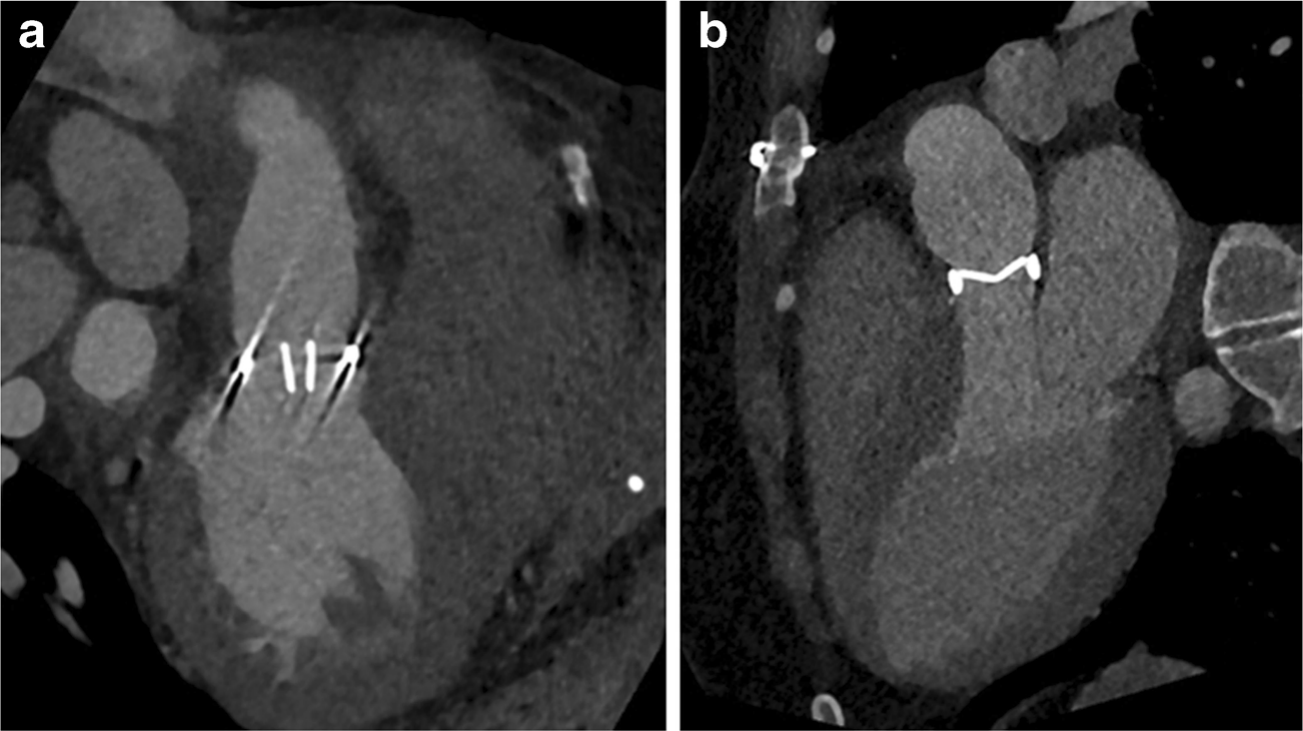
Image quality. Example of CT angiography (CTA) reconstruction in systole and diastole showing excellent image quality of a bi-leaflet prosthetic heart valve in the aortic position with valve in open (A) and closed (B) position
